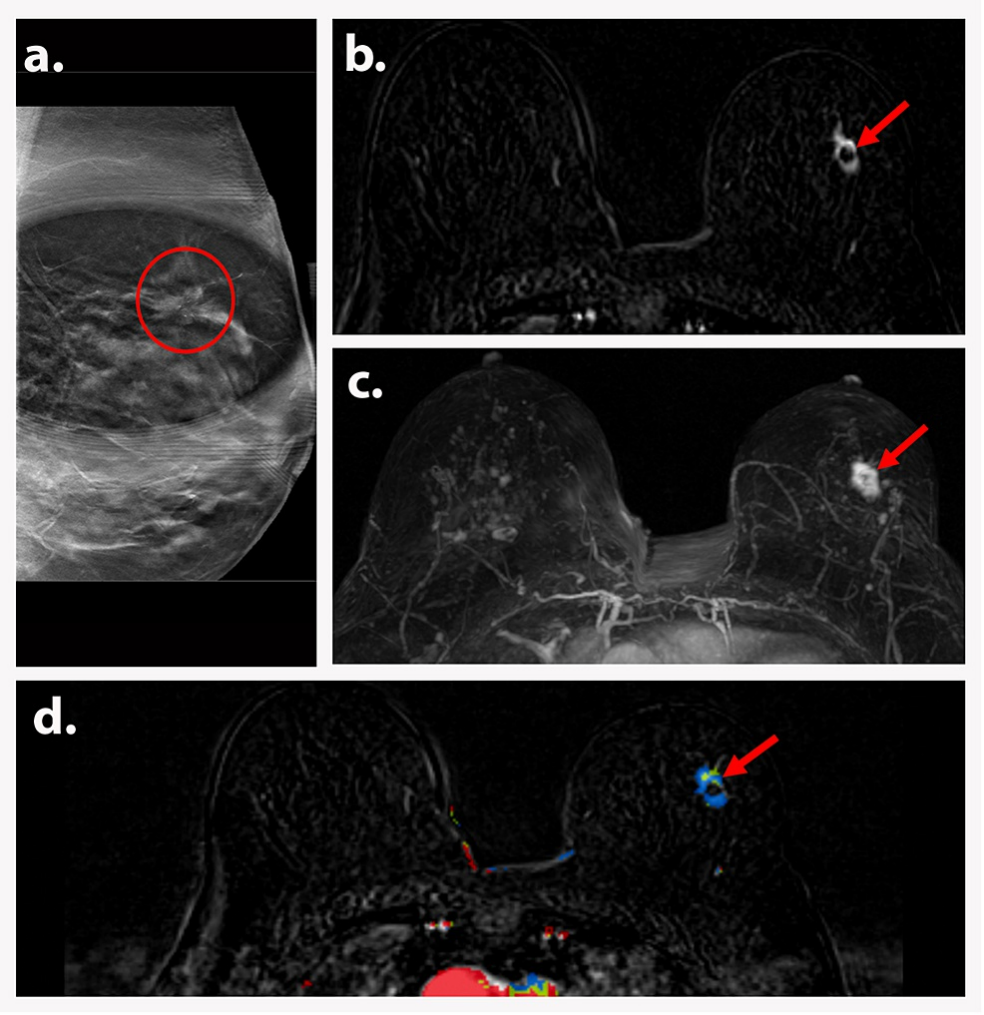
AJCC TNM stage IA (T1cN0M0) A 56-year-old postmenopausal woman presented with suspicious left breast mass on a screening mammogram. Left MLO spot compression view on a diagnostic mammogram (a) shows an irregular mass with spiculated margins (red circle) in the superior left breast. Axial T1-weighted DCE subtraction image (b) shows a 2 cm irregular enhancing mass (red arrow) with susceptibility artifact from biopsy clip without ipsilateral axillary lymphadenopathy. Axial MIP (c) and axial T1-weighted DCE with color (d) images demonstrate the irregular enhancing mass with persistent kinetics (red arrows). Biopsy depicted invasive carcinoma. Findings are consistent with AJCC TNM stage IA (T1cN0M0). AJCC: American Joint Committee on Cancer; TNM: tumor, node, and metastasis; MLO: mediolateral oblique; DCE: dynamic contrast-enhanced; MIP: maximum intensity projection.
